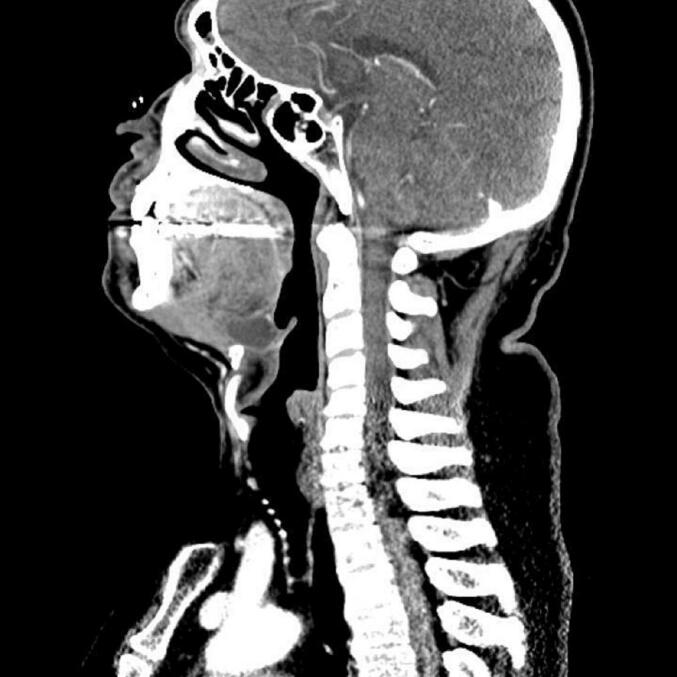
Sagittal, contrast-enhanced computed tomography (CT) image of Patient 1 demonstrates a cystic lesion above the hyoid bone.
